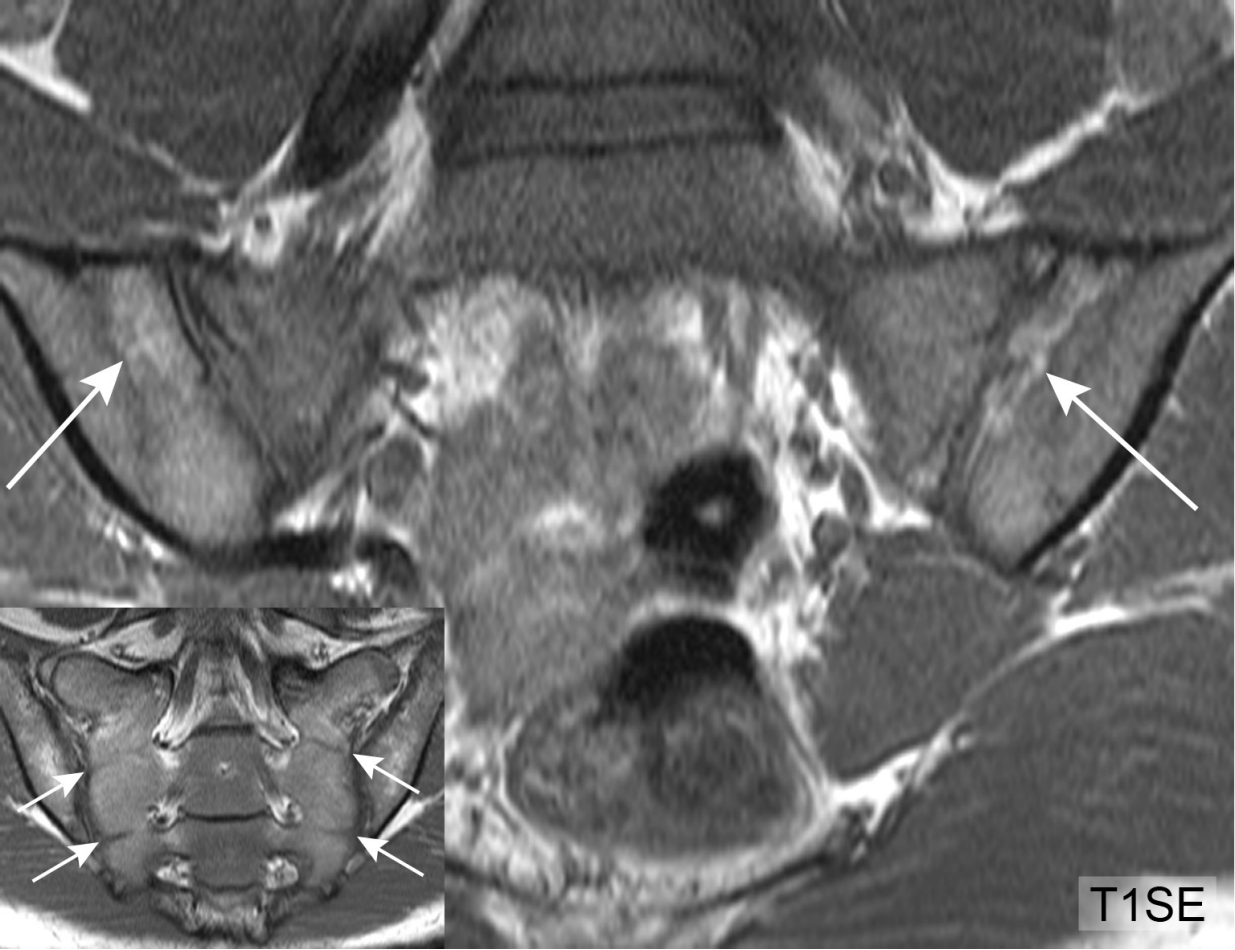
Open epiphyseal growth plate mimicking backfill (T1SE sequence). 18-year-old male healthy control. Irregularly shaped increased signal alteration on T1SE sequence (arrows) compatible with fat infiltration of the anterior predominantly iliac joint portion. Insert: The sacral vertebrae are not yet completely fused (arrows). T1SE, T1-weighted spin echo sequence.
